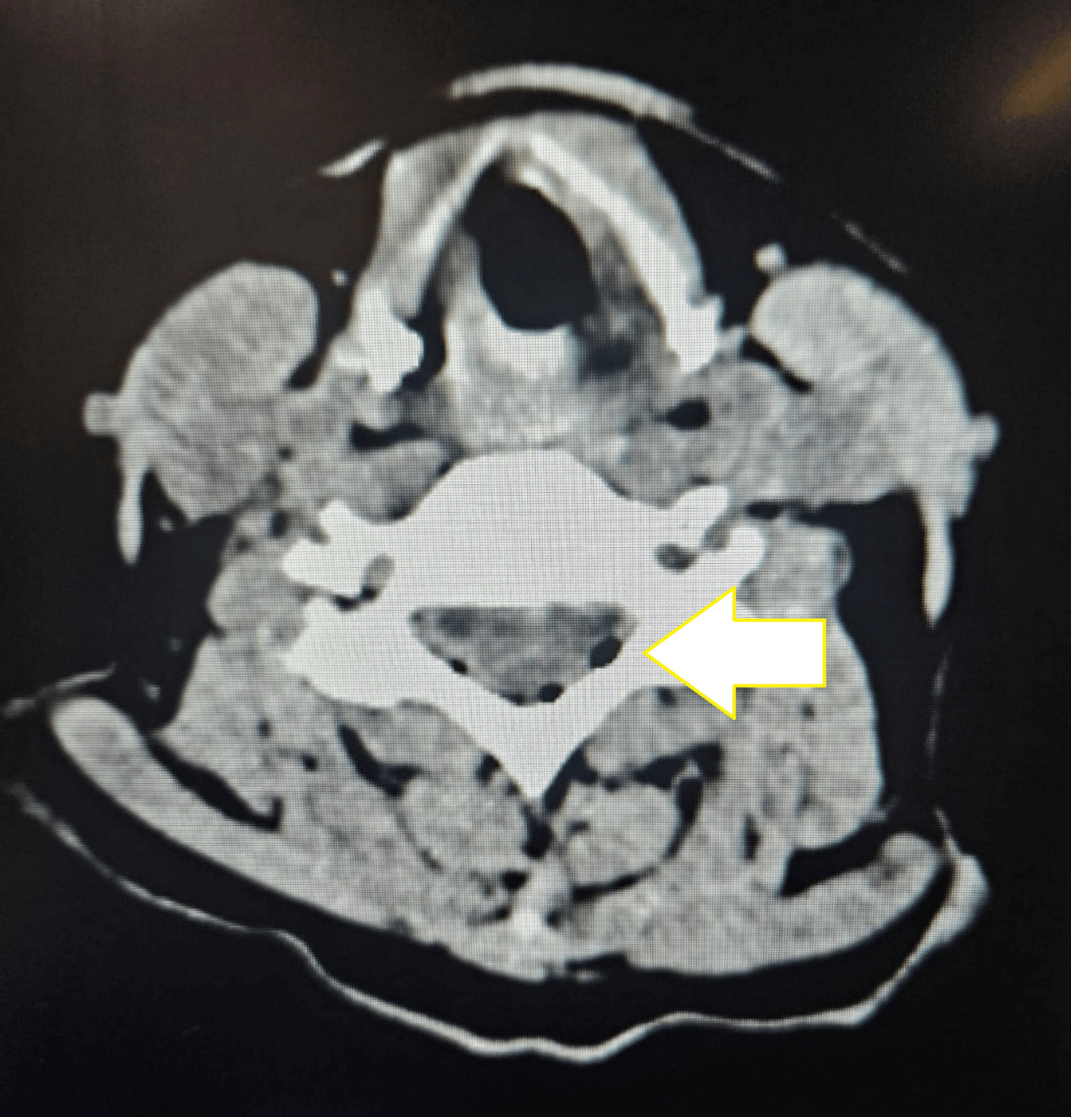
Non-contrast CT of the brain (axial plane) showing cervical extradural pneumorrhachis (C1–C4) with associated retropharyngeal and paravertebral air locules. Pneumorrhacis is indicated by the white arrow.
